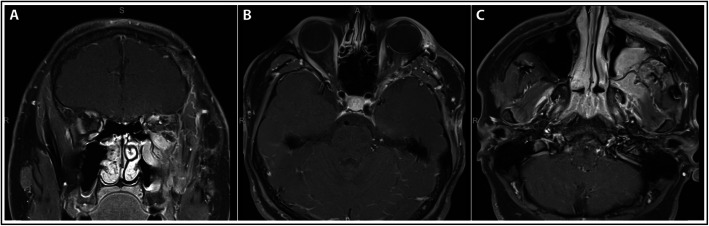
Follow-up MRI brain and orbits after second surgical debulking. Follow-up T1-weighted post-contrast MRI brain and orbits obtained approximately 4 months after second surgical debulking showing stable residual disease in the superior left maxillary sinus and along the left orbital floor involving the inferior rectus muscle in the (a) coronal plane, (b) axial plane at the level of the optic nerve, and (c) axial plane at the level of the maxillary sinus. Notably, the globes are normal in contour and there is resolution of prior proptosis
